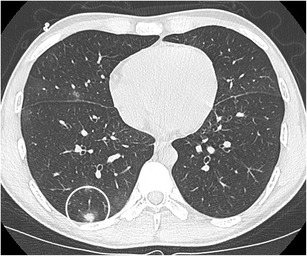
Axial CT-image in lung window setting in a 25-year-old immunocompromised man with a myelodysplastic syndrome treated with induction chemotherapy shows a subpleural nodule in the right lower lobe. The nodule has a predominant solid aspect with surrounding ground glass halo. Also note the small foci of ground glass in the right lung. Clinical and laboratory findings were consistent with invasive aspergillosis
